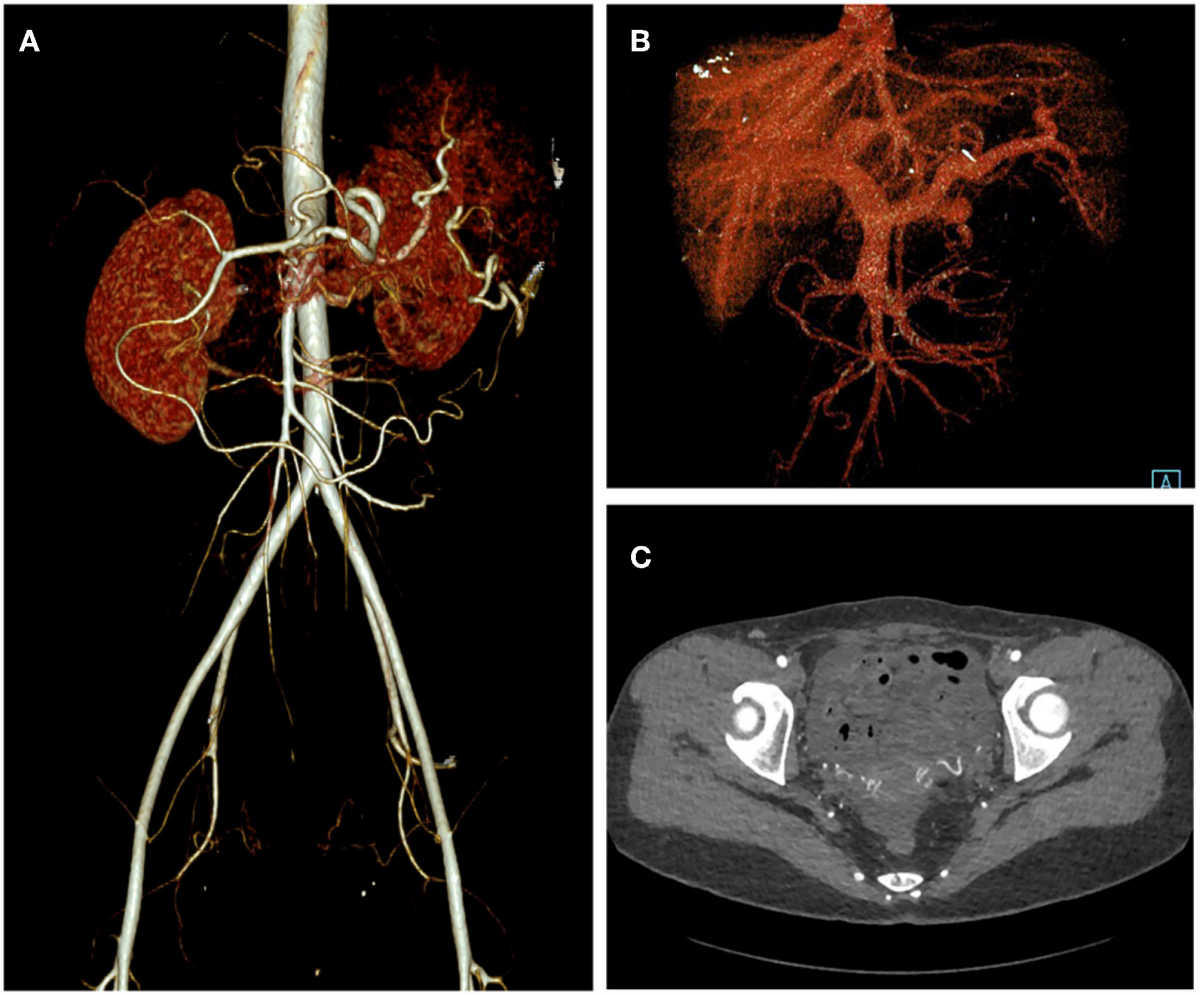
One-month post-operative CT angiography of the patient. Arterial phase (A), Venous phase (B), and axial view (C) of the CT angiography showed elimination of the abdominopelvic AVM.
